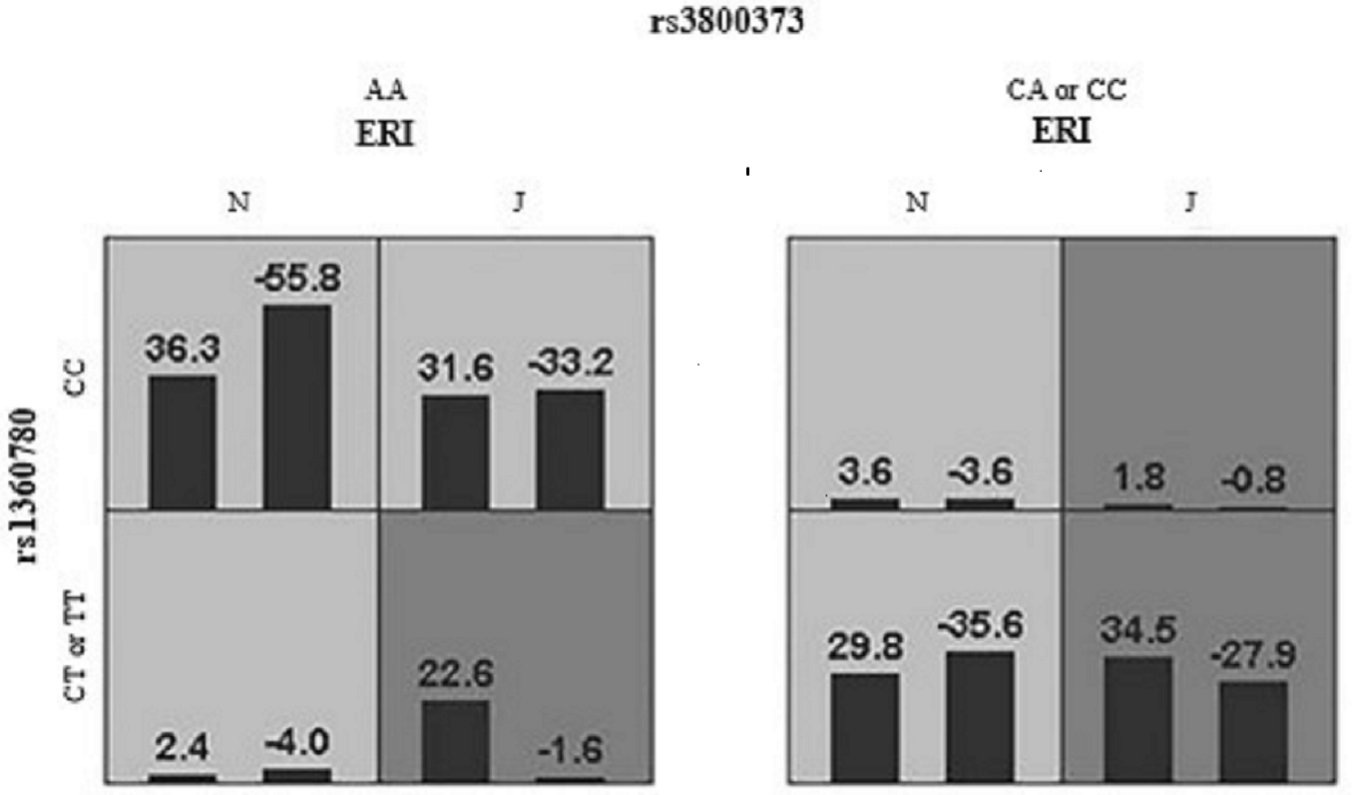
The GMDR results of the interaction between FKBP5 gene and job stress. High- and low- risk factors are inherently determined by the model, in which the dark gray box represents the high-risk factors, and the light gray represents the low-risk factors. Bars represent the maximum likelihood estimation of case weights. In the same box. the left column is positive score and the right column is negative score. N and J denote normal and job stress (ERI>1), respectively. Among them, individuals with job stress, CA or CC genotype of rs3800373 and CT or TT genotype of rs1360780 had the highest sum score.
